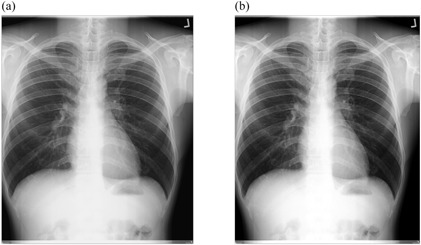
High kVp chest radiograph: (a) original image and (b) processed image by background subtraction and pixel adjustment.
